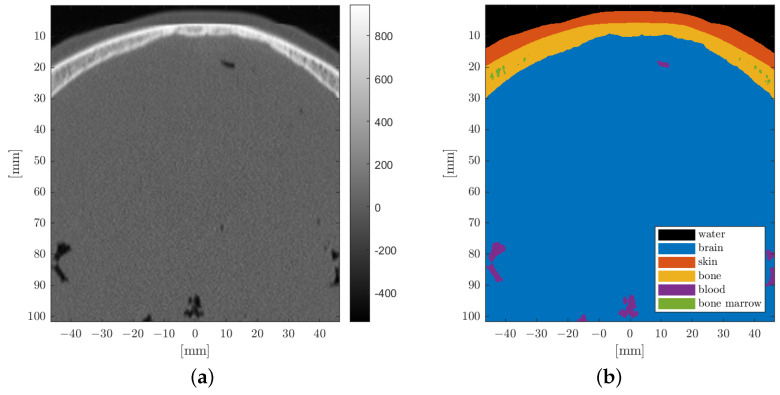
Cranial section of the CT image (a) and corresponding segmentation into the predominant tissue types (b). (a) CT, Hounsfield unit. (b) CT, clustered.
